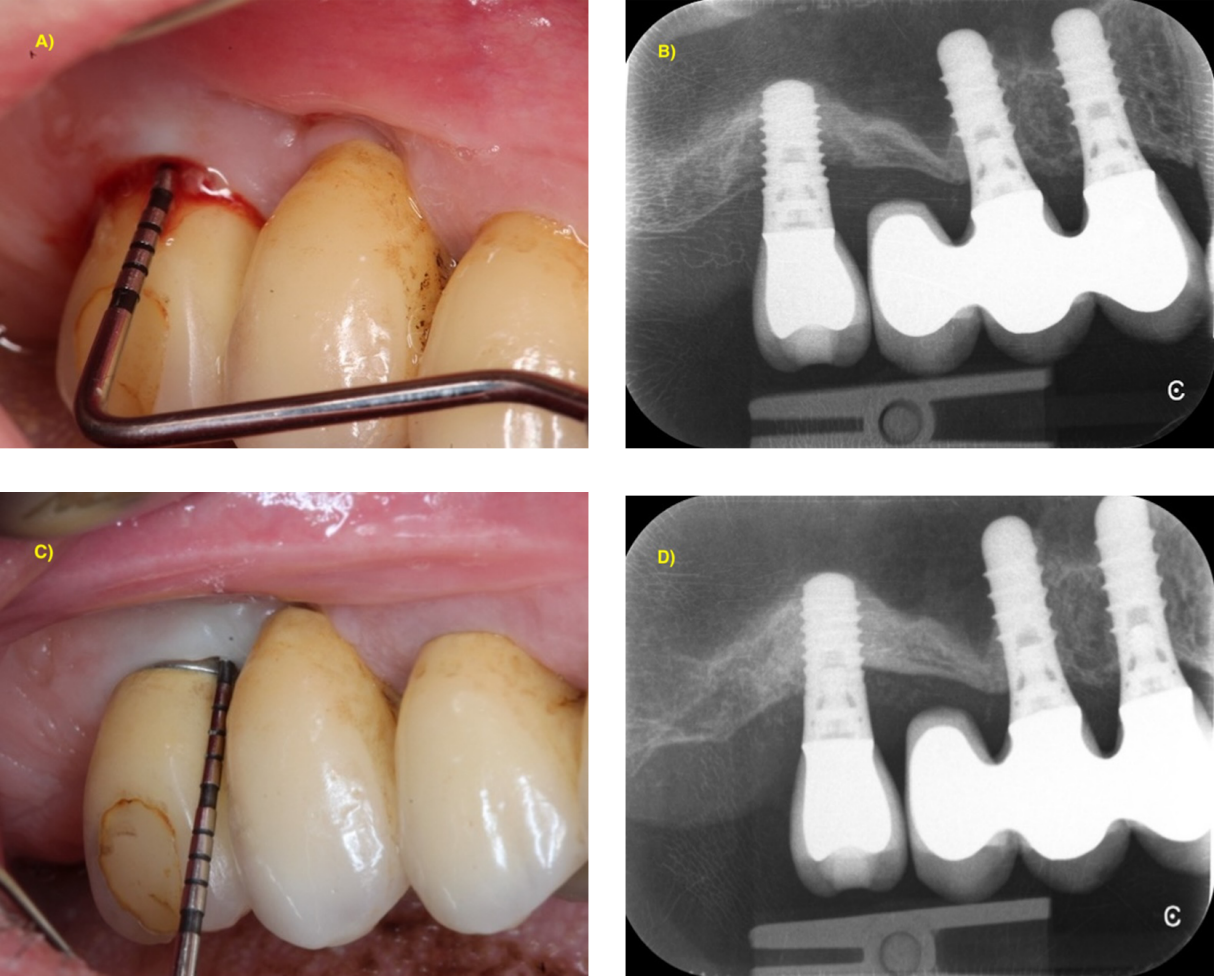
1-year stabilization of advanced peri-implantitis showing BL > 50% of implant length with combined surgical approach (reconstructive + implantoplasty). (A) Baseline clinical situation with PPD = 8 mm and BOP; (B) periapical x-ray showing intrabony bone loss up to the apical third of the implant; (C) clinical situation at 1-year post-operative follow-up with PPD = 4 mm without BOP; (D) periapical x-ray showing complete bone filling of the intrabony component.
